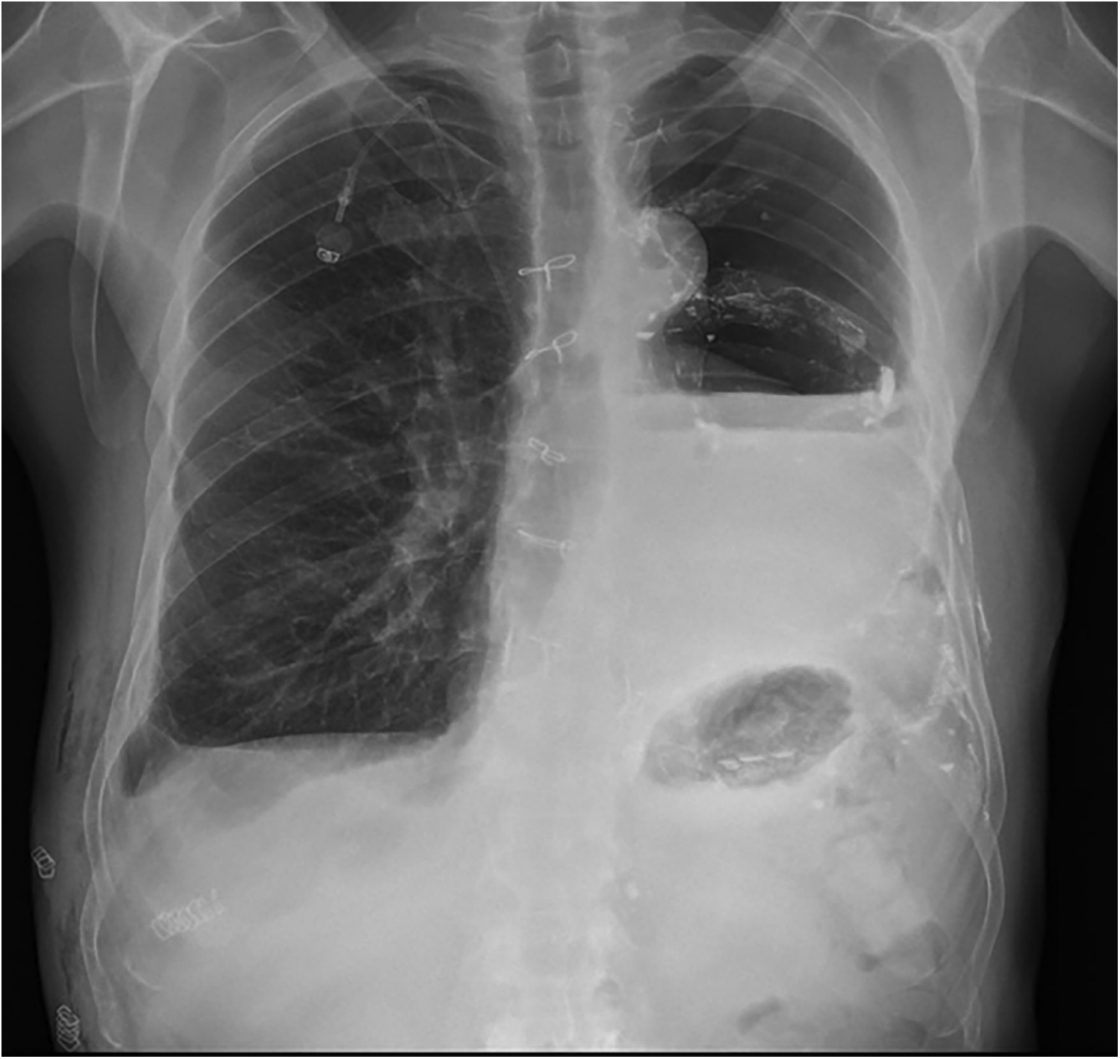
Control chest x-ray before discharge.
